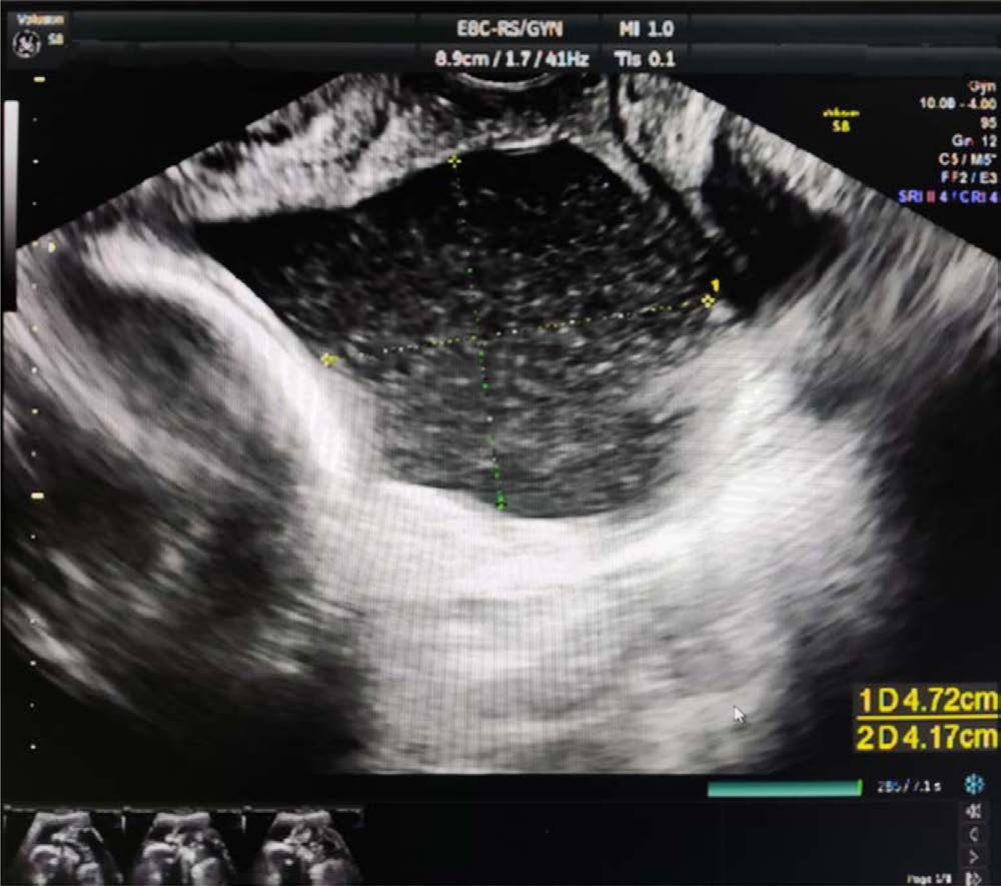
TVS of cervix at 21 + 6 weeks of pregnancy. TVS = transvaginal sonography.
